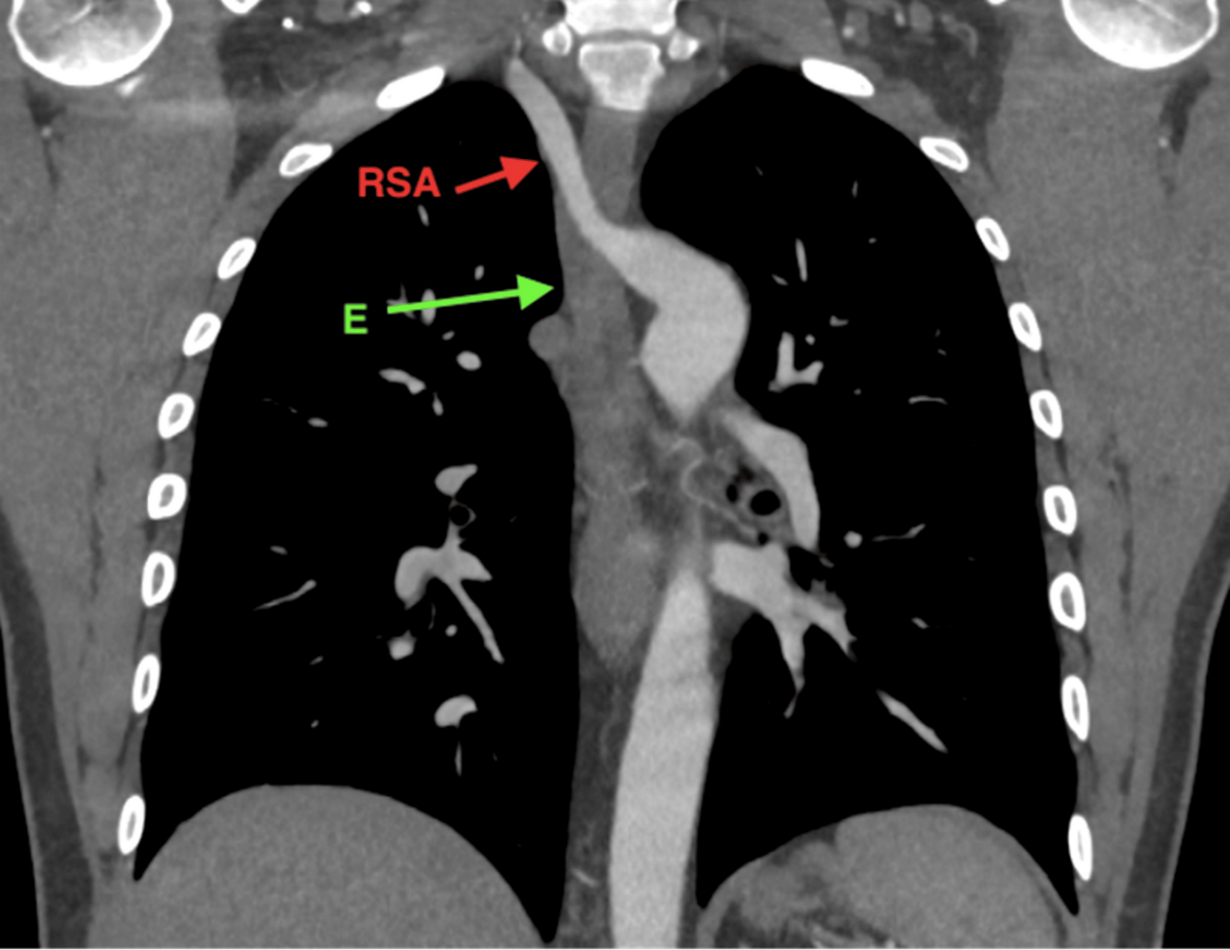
CT angiography: coronal section of the thorax at the level of the esophagus. The ascending course of the RSA from the left to the right is visualized, originating from the posterior wall of the distal aortic arch. Note the slight enlargement at the origin of the RSA. CT: computed tomography; E: esophagus; RSA, right subclavian artery.
